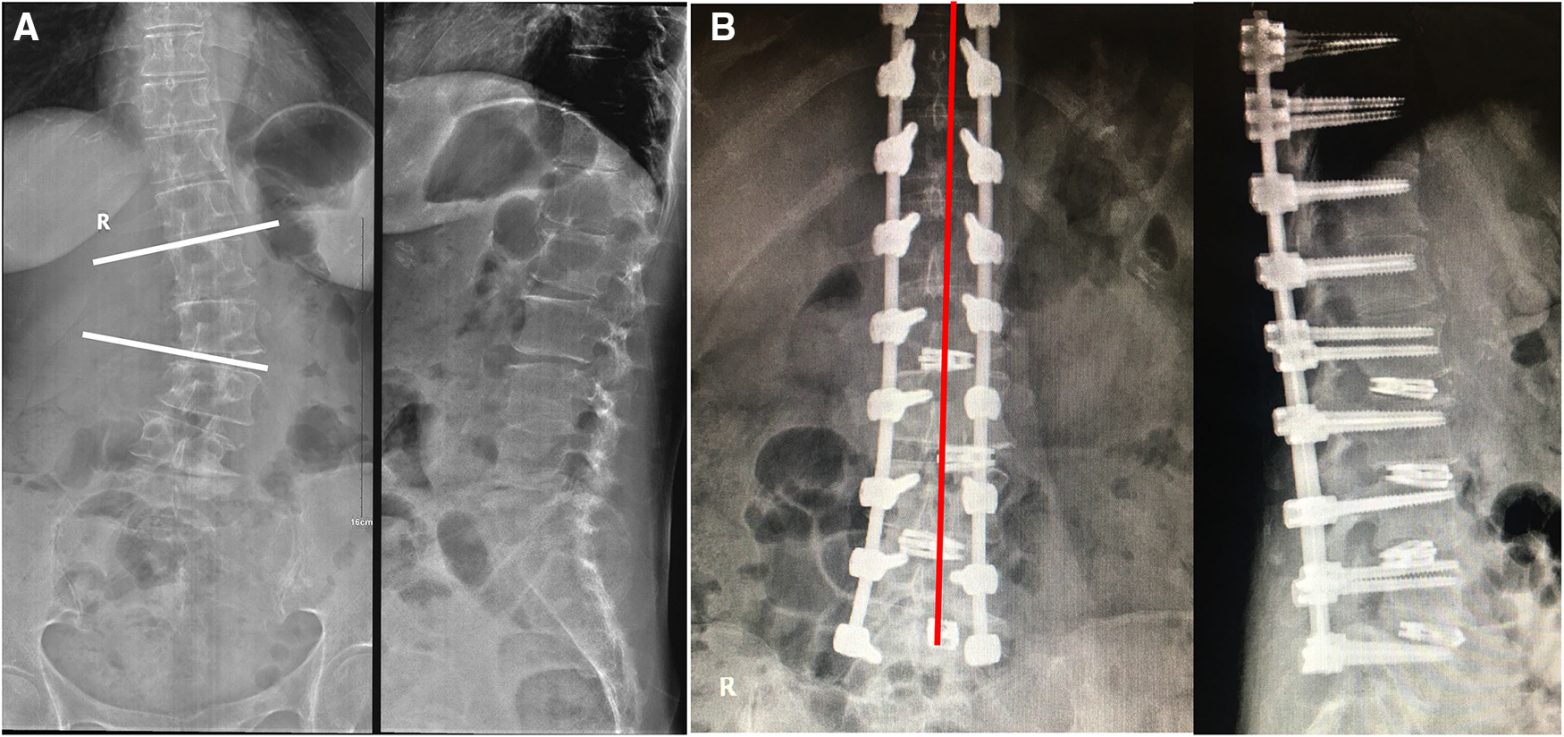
X-rays of the 61-year-old female patient with ADS, which was taken on December 2018: (A) before operation and (B) after operation.
